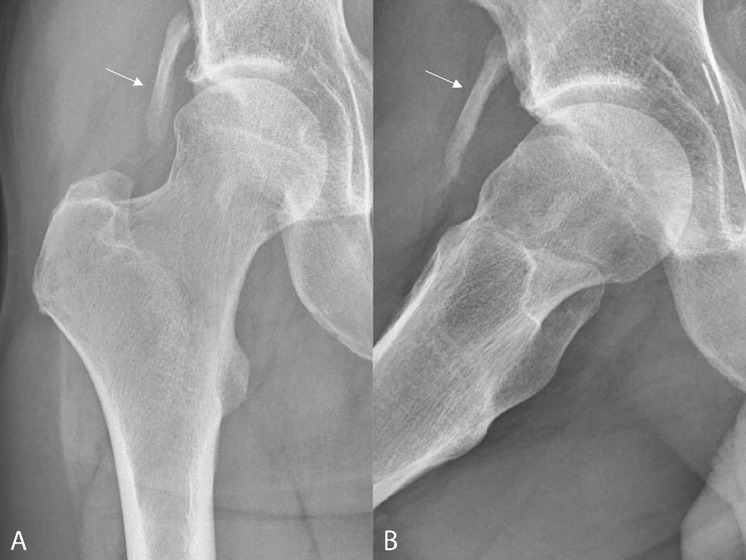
Conventional radiography shows an elongated, well circumscribed heterotopic ossification projecting superolaterally to the anterior inferior iliac spine (AIIS) (arrows). There is also cam deformity with loss of concavity of the femoral head‑neck junction.
